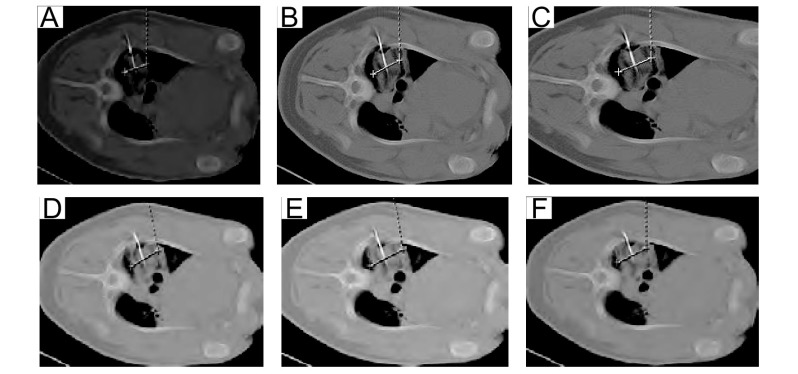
冷冻过程中（3个循环）冰球的CT表现 Sequential changes of the pulmonary parenchyma on computed tomographic scanning after freezing and thawing (3 cycles). A: 5 min after the beginning of first freezing; B: 5 min after the beginning of first thrawing; C: 5 min after the beginning of second freezing; D: 5 min after the beginning of second thrawing; E: 10 min after the beginning of third freezing; F: 5 min after the beginning of third thawing.
